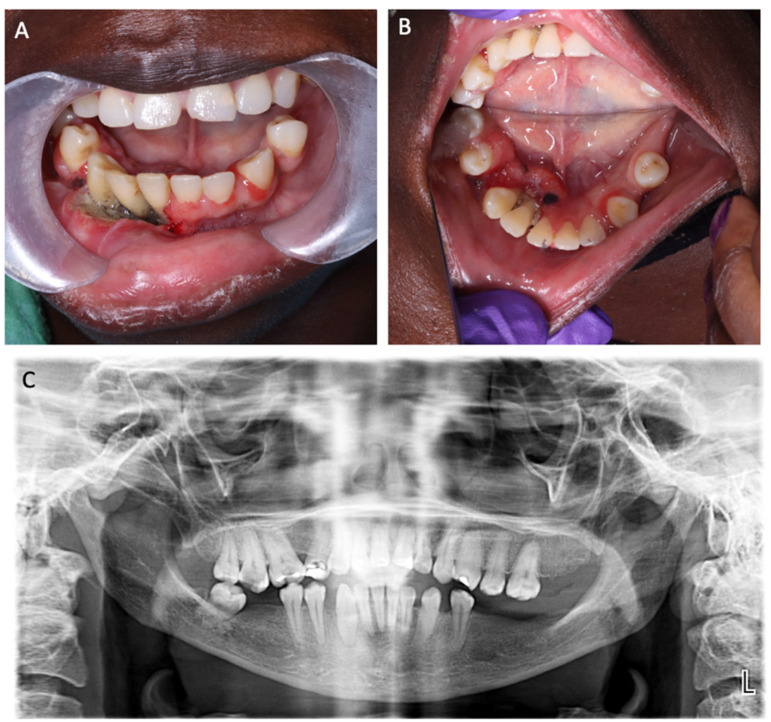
(A,B) Preoperative clinical situation of case 2. (C) Preoperative orthopantomogram of case 2.
